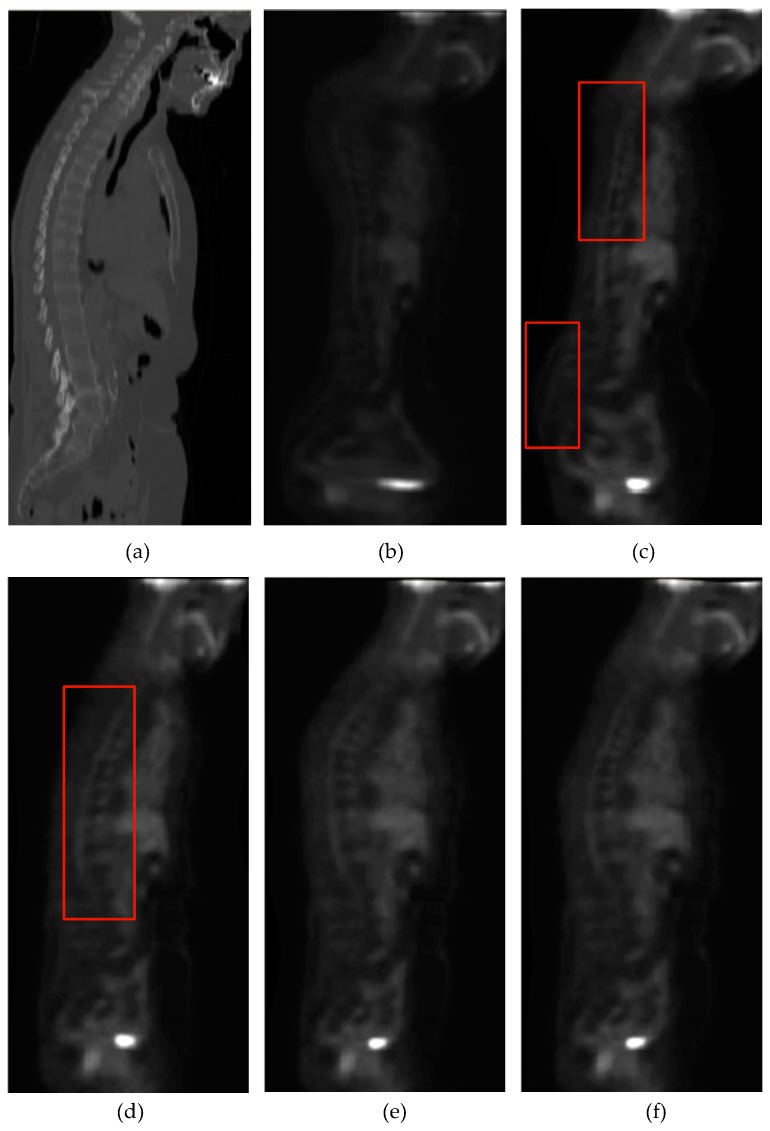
Registration results of all evaluated methods operating on the 3D CT–PET images. (a) CT (reference image); (b) PET (float image); (c) ESSD; (d) MIND; (e) HLCSO; and, (f) FMIND.
